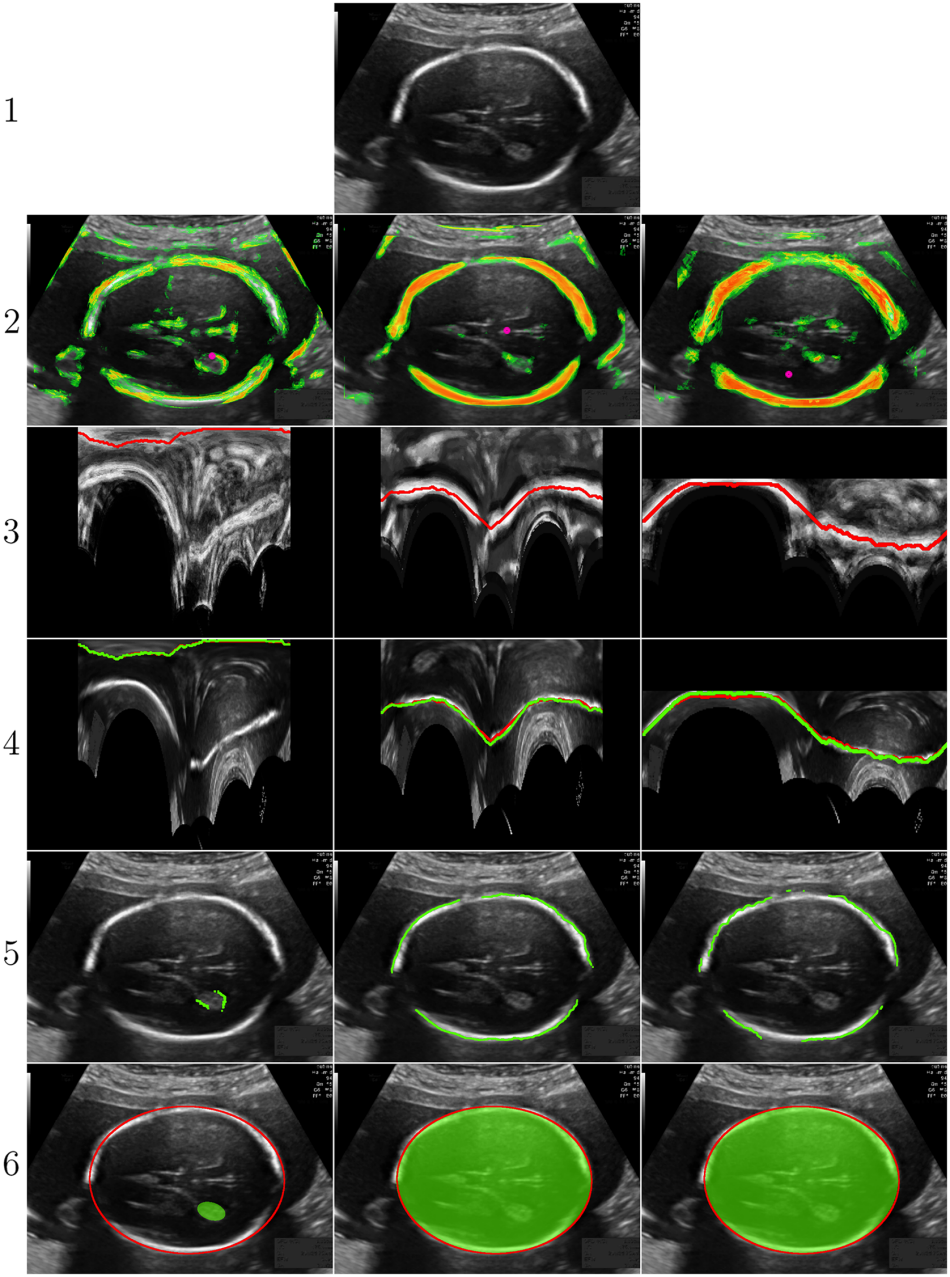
Steps of quantification system *C*. From left to right: pipeline 1, 2, and 3, respectively. From top to bottom: (1) Input image. (2) Input ultrasound image with overlay of pixel classifier likelihood ranging from green to red and Hough transform result in pink. (3) Polar transformed pixel classifier likelihood with overlay of dynamic programming in red. (4) Polar transformed ultrasound image with overlay of dynamic programming in red and repositioned dynamic programming result in green. (5) Ultrasound image with overlay of the highest five percentile repositioned dynamic programming pixels. (6) Ultrasound image with fitted ellipse in green and annotation of the experienced sonographer in red. In this example image, the pipeline that was optimized for the second trimester is automatically selected as the best result, since the edge of this fitted ellipse has the highest median pixel classifier output.
